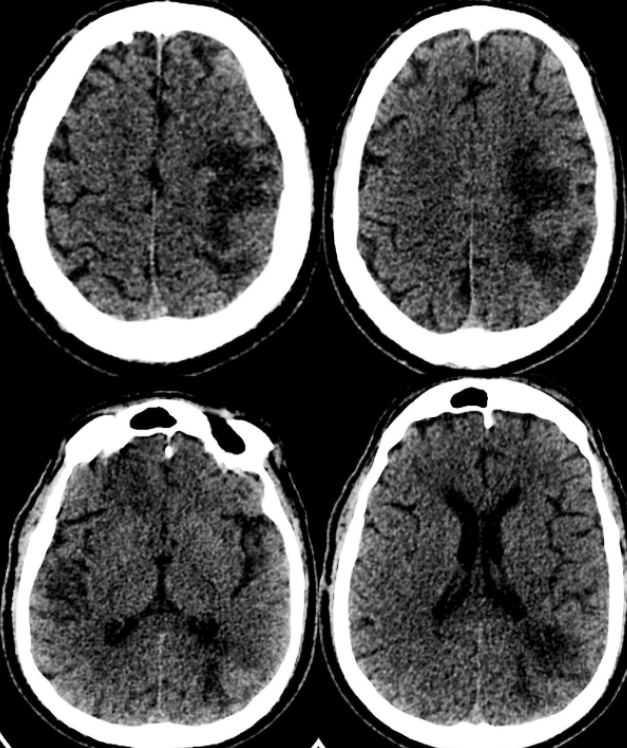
Computed Tomography of the skull without contrast showing loss of differentiation between the white-gray substance, hypoattenuation of deep nuclei, and an extensive area of the vascular territory of the left middle cerebral artery.
